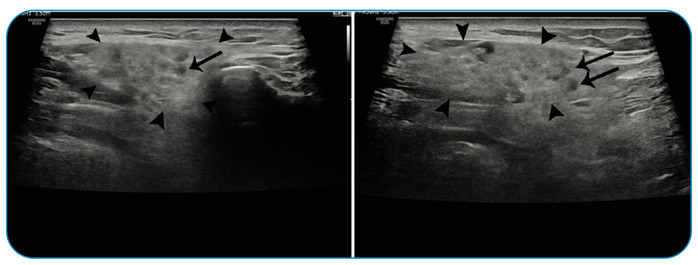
Sonographic examination showed increased echogenicity and thickness of the right parotid gland (arrowheads). Hypoechoic areas (arrows) were noted within the gland, which were consistent with the features of parotitis.
